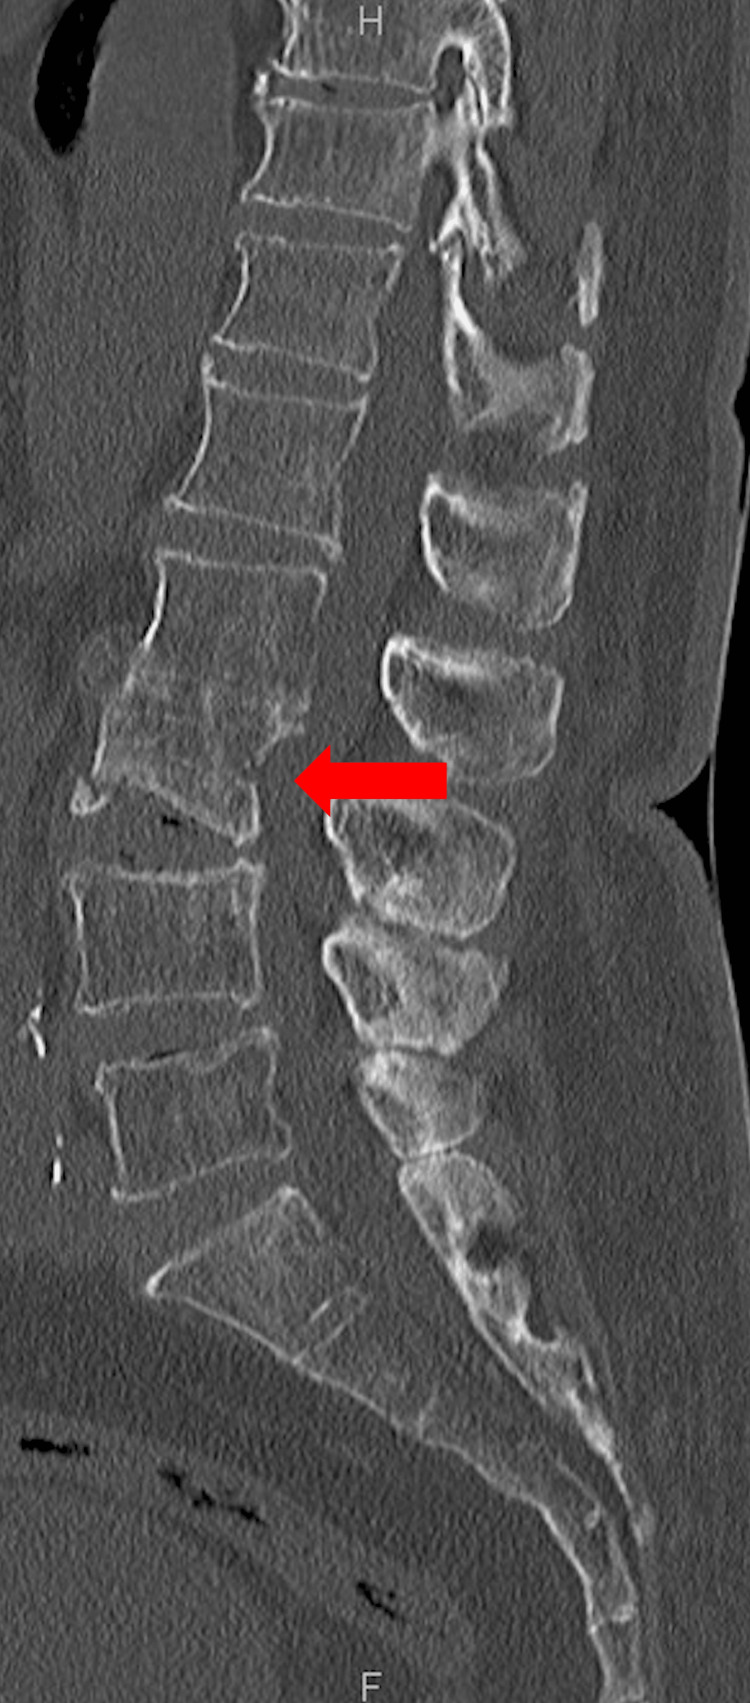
Computed tomography of the lumbar spine on arrival showed an L3 fracture (red arrow)
